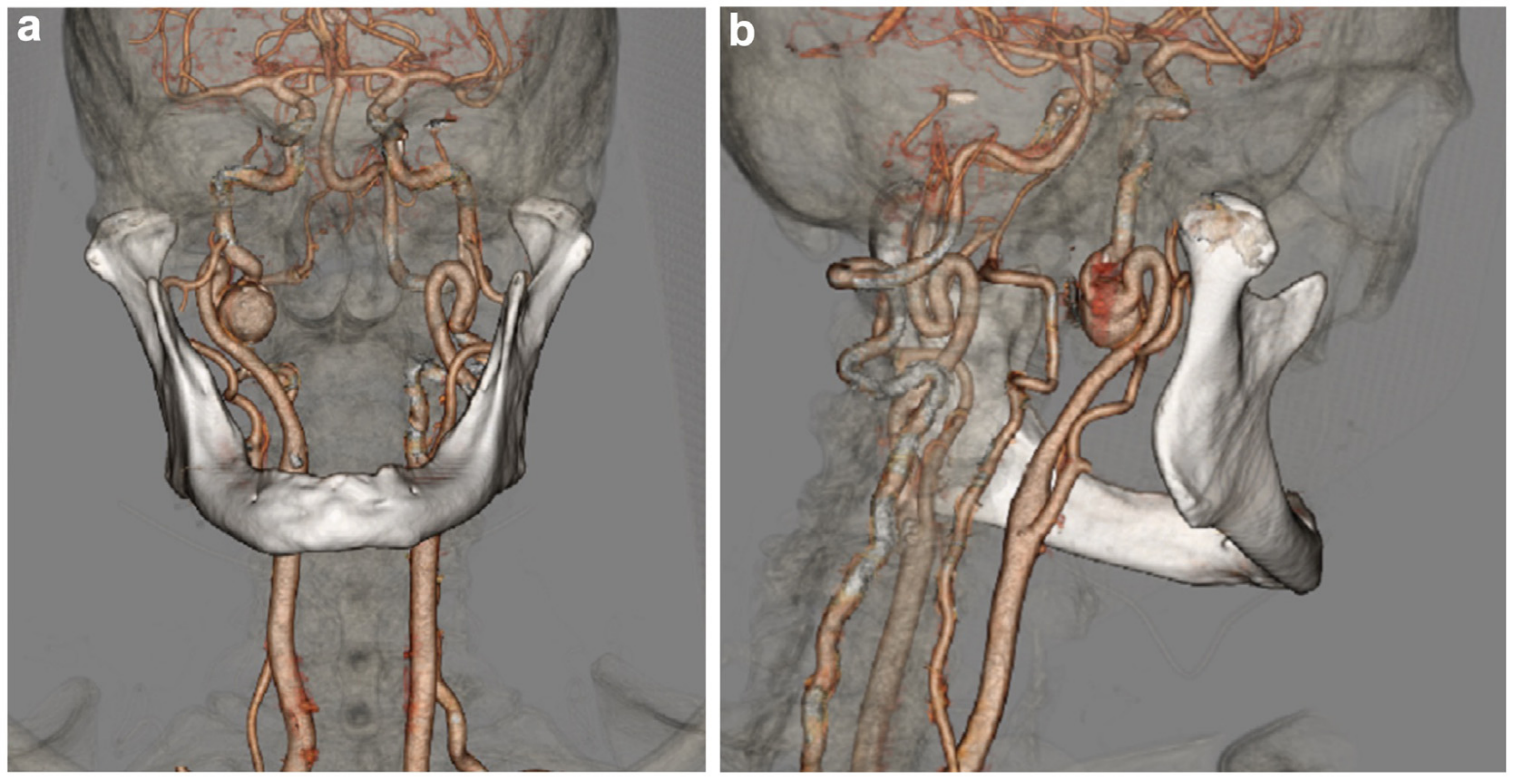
Three-dimensional computed tomography images of the aneurysm. A, Anterior view. B, Posterolateral right view.
